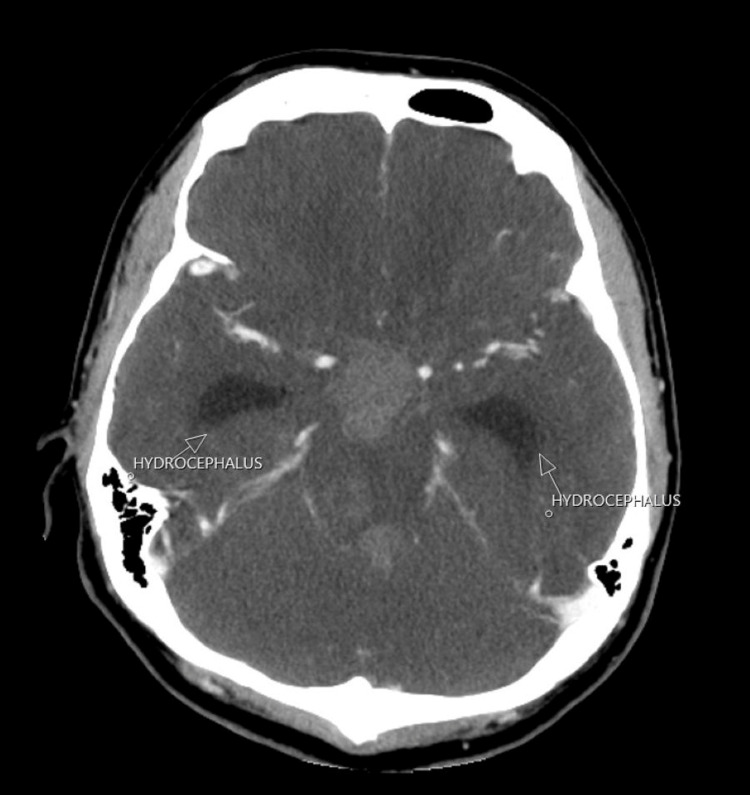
CT of the pituitary with contrast. White arrows: hydrocephalus.
